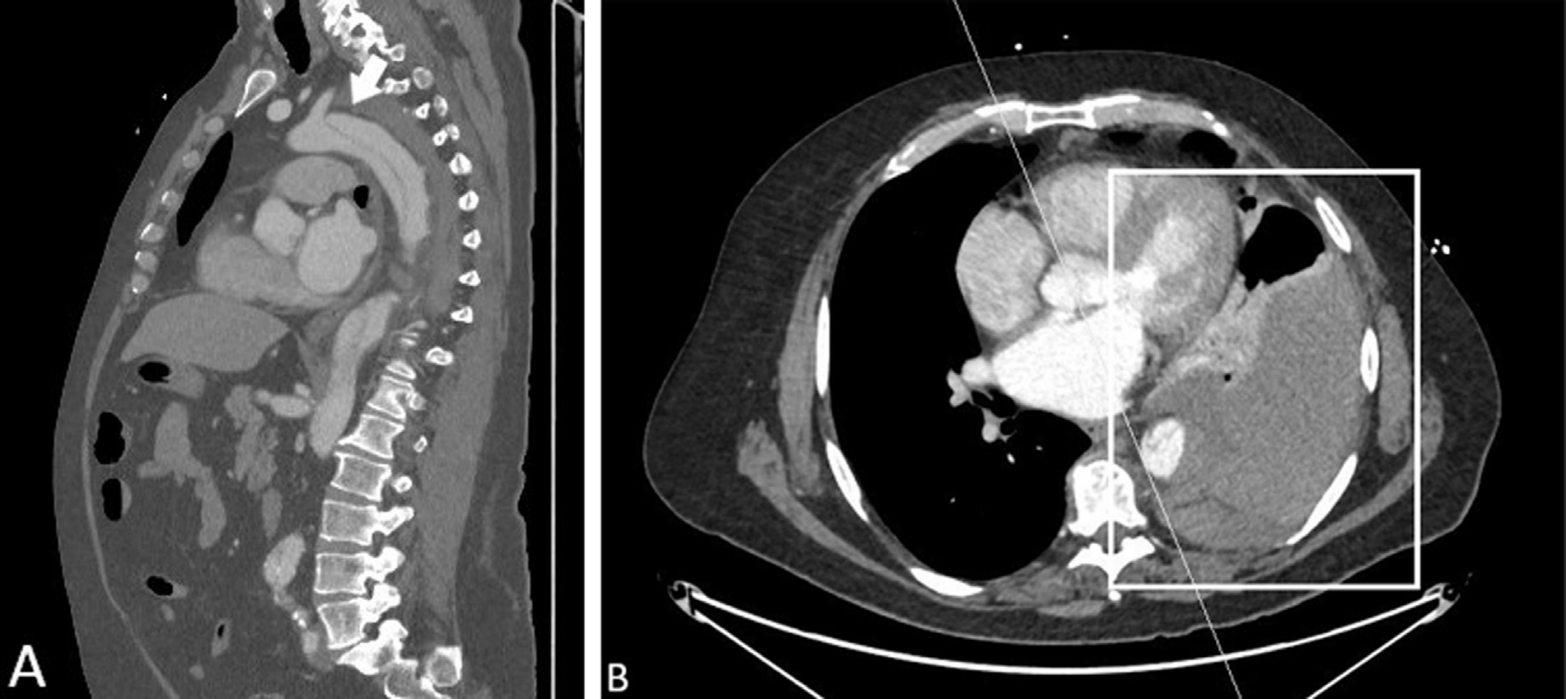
Computed tomography angiography images at initial presentation. (
